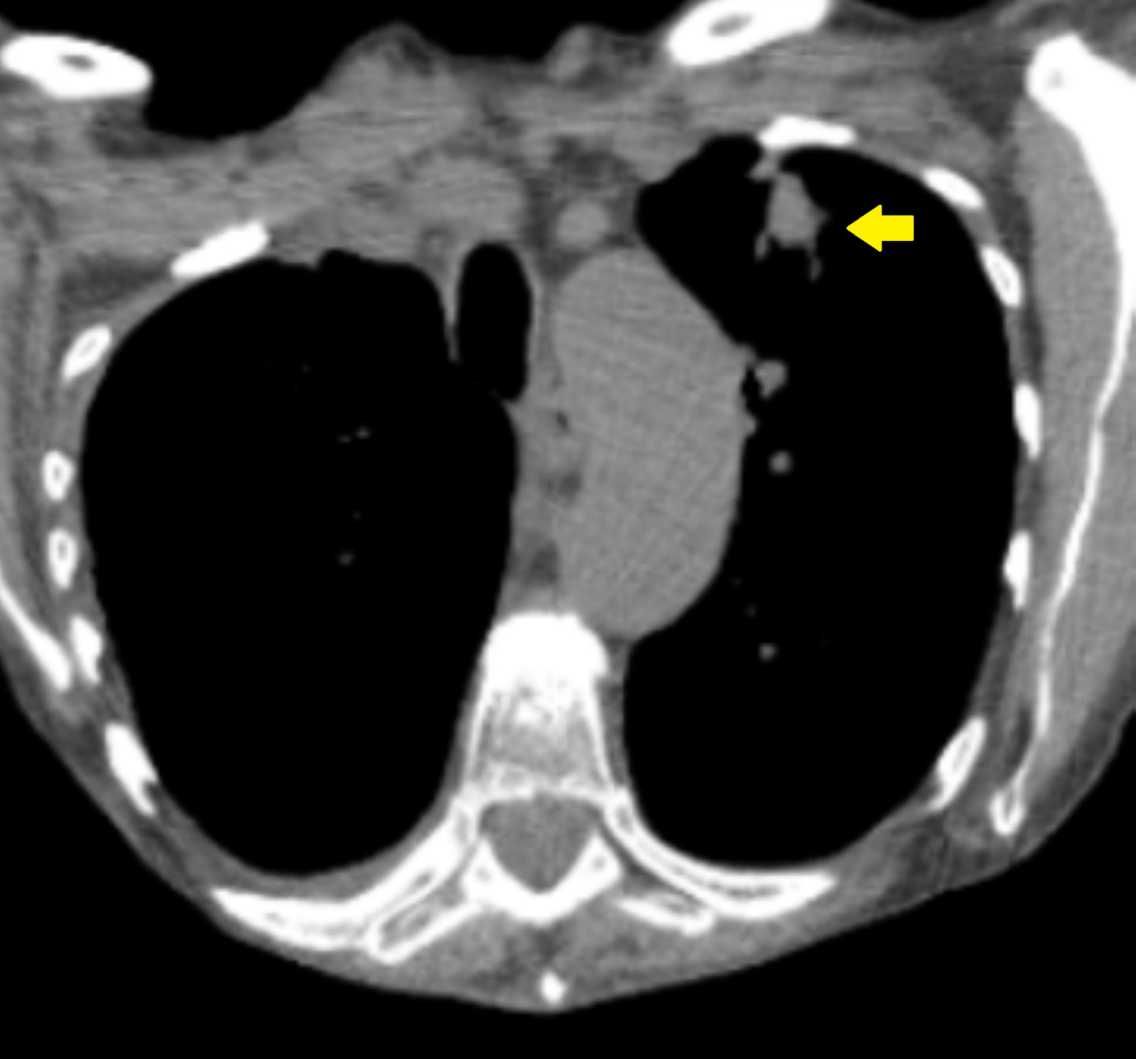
CT of the chest The yellow arrow pointing towards a lesion in the left upper lobe of the lung that is approximately 2.8-3.0 cm in diameter.
